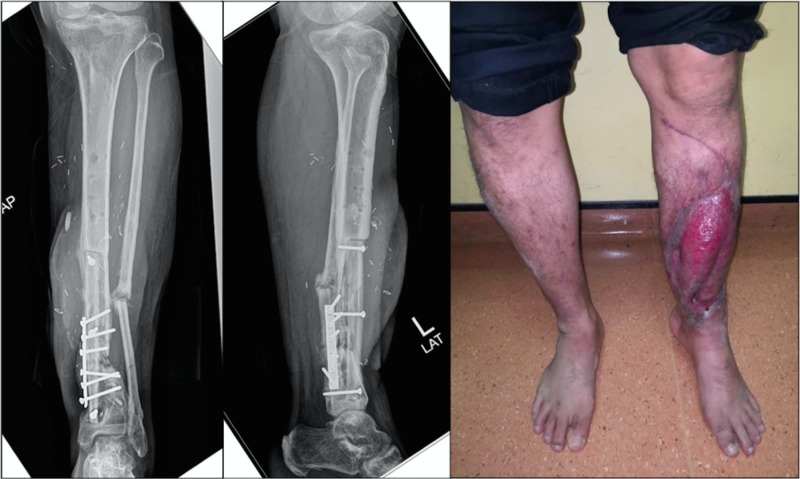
Radiographs at six months post-operatively showed viable vascularized fibula bone graft with callus formation around fracture sites. The patient is able to perform partial weight bearing ambulation with crutches.
